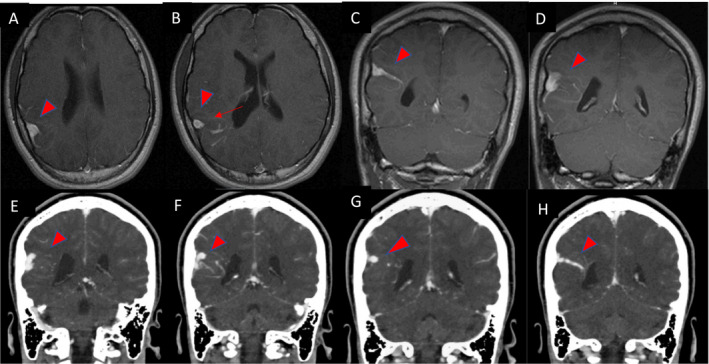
Axial postcontrast T1W MRI demonstrating a typical dural venous anomaly (arrowheads) (A,B,C,D) with a “medusa’s head (arrow) (B). Coronal images from a CT with venous phase, demonstrating the dural venous anomaly (arrowheads) extending from the ependymal surface to the cortical surface (F,G,H,I).
